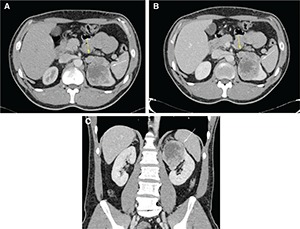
CT: axial scans during arterial (A) and venous (B) phases and coronal scan during venous phase (C) of the abdomen, show expansive lesion, partially exophytic, located in the upper pole of the left kidney (diameters 72 × 56 × 70 mm) with necrosis in the context (white arrows); it also observes repetitive lesion of the right adrenal gland body (diameter 20 mm) (A and B) (yellow arrows).
